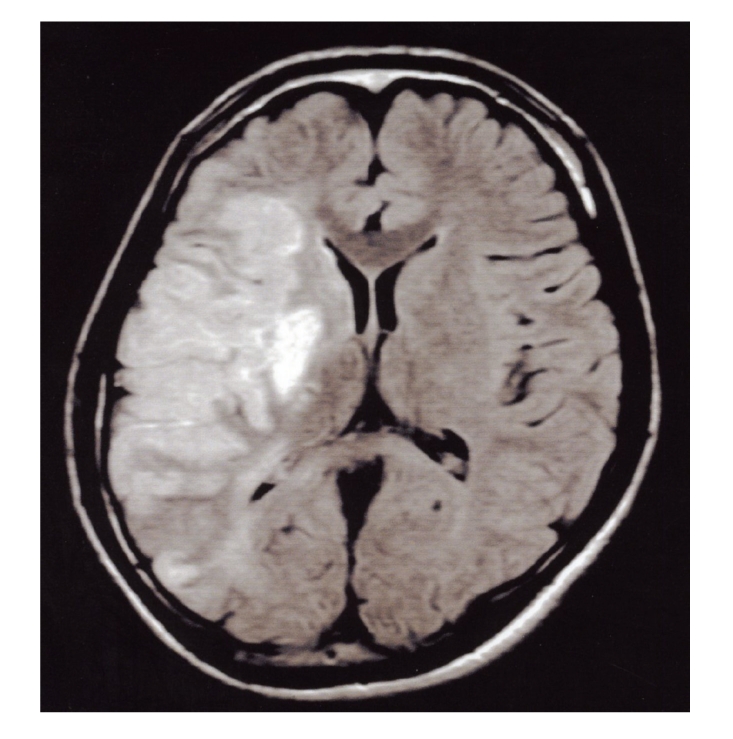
Axial fluid-attenuated inversion recovery magnetic resonance image showing cerebral infarction in the right cerebral hemisphere.
